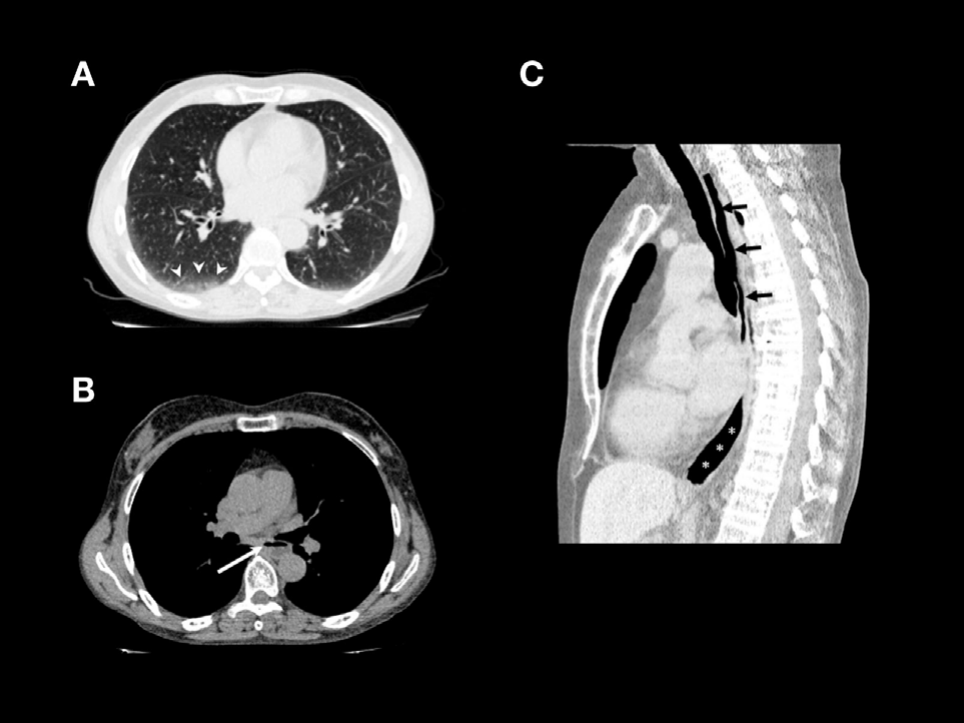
(A) Non-contrast chest CT (lung window) shows interlobular septal thickening and ground-glass opacities in the immediate subpleural lung with peripheral and lower lobe predominance, especially on the right side (arrowheads). (B) Non-contrast chest CT (mediastinal window): air–fluid level due to stasis in thoracic esophagus (arrow). (C) Non-constrast chest CT, sagittal reformation: air-filled hypotonic upper (arrows) and lower (asterisks) thoracic esophagus.
